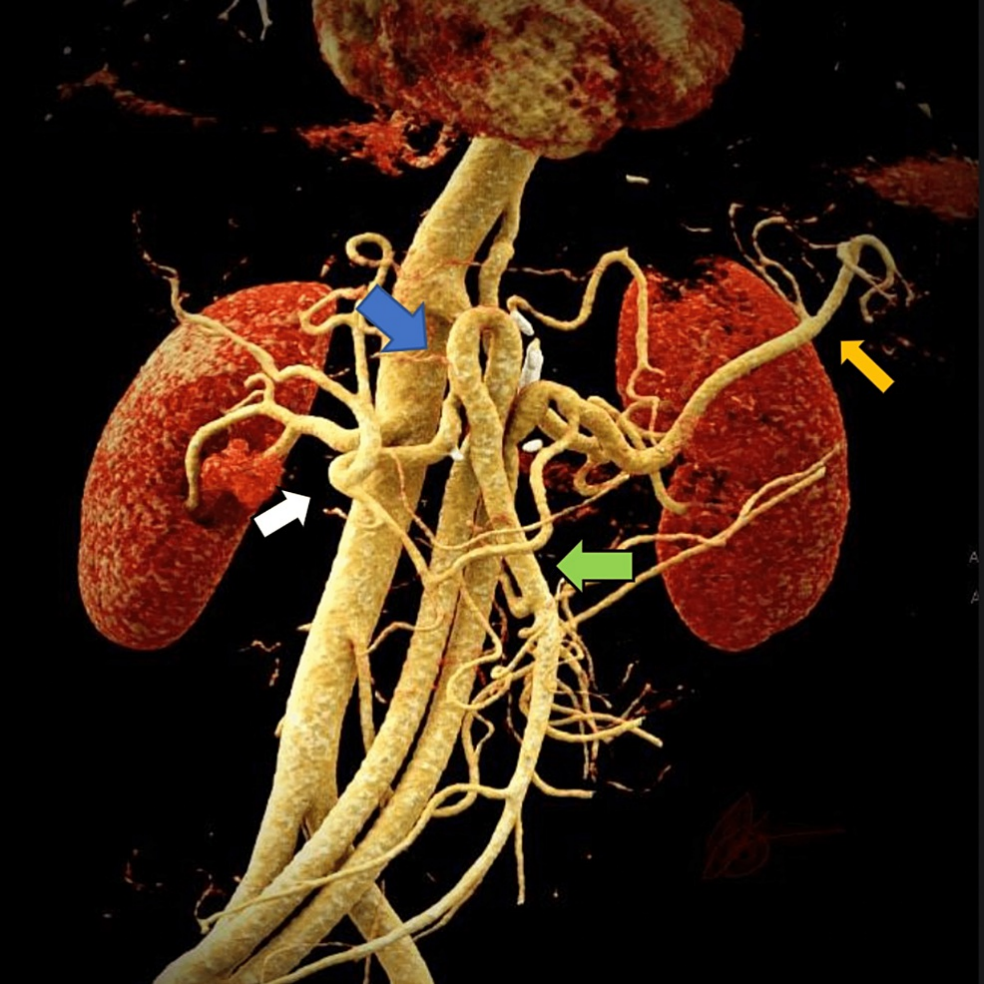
Postoperative 3D reconstruction and cinematic rendering of the CTA highlighting the debranching to the splenic artery (orange arrow) and the common origin (blue arrow) of the common hepatic (white arrow) and superior mesenteric (green arrow) arteries CTA: computed tomography angiography
